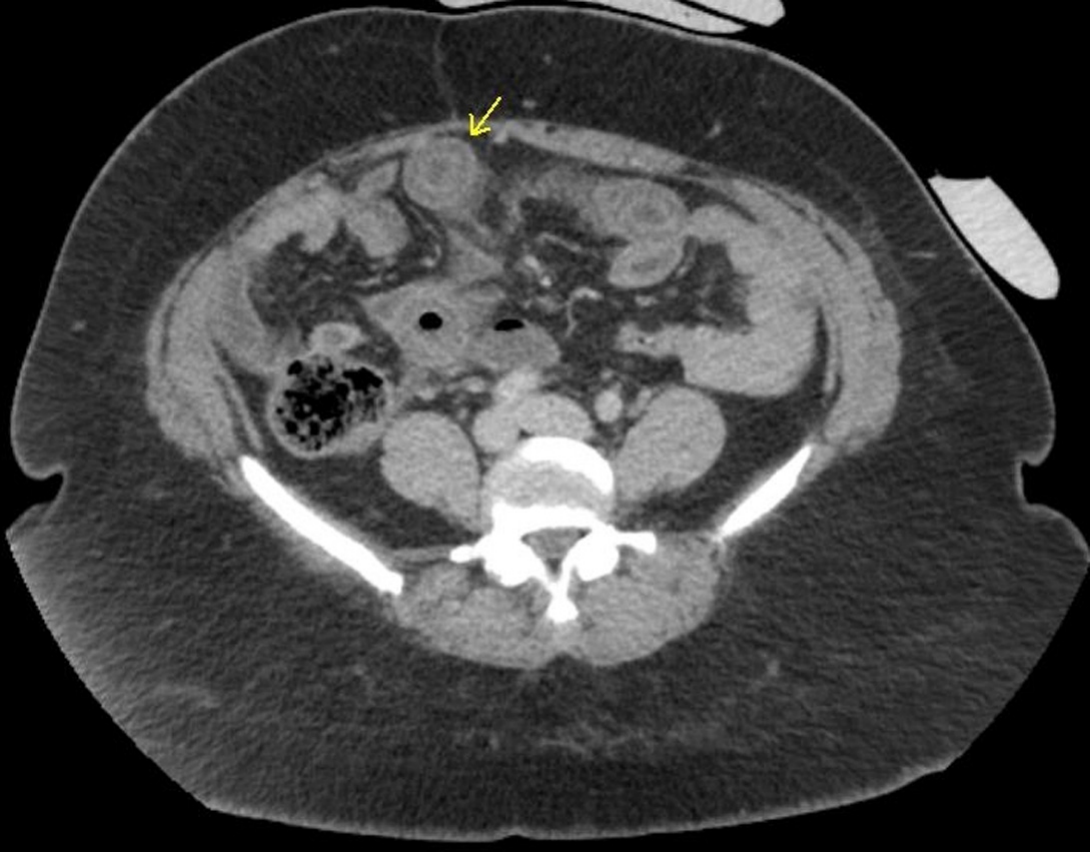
Axial contrast‐enhanced CT images demonstrated thickening of the bowel wall with submucosal edema and all layers of the bowel delineated. (Yellow arrows).
